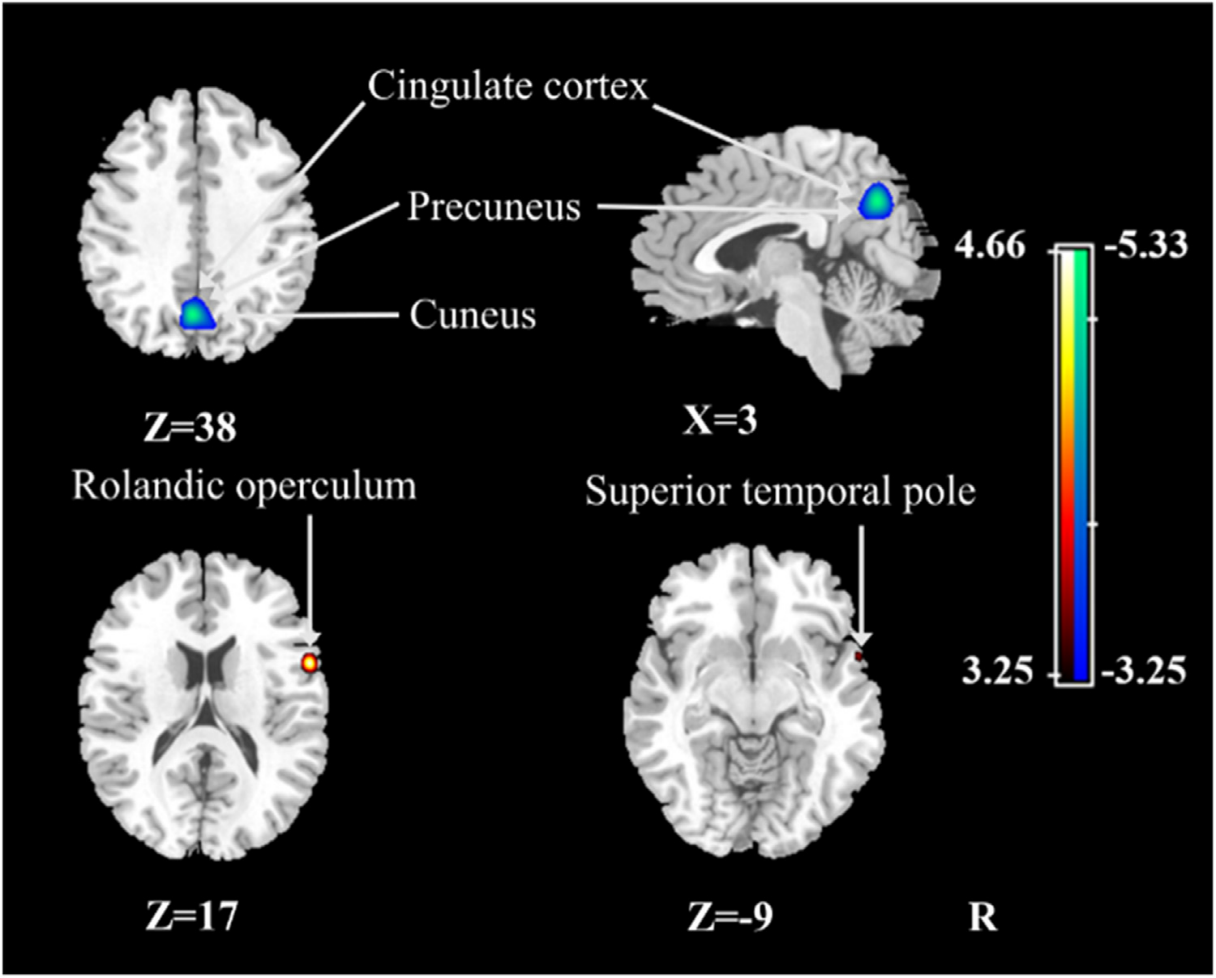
Regional changes in relative CBF after ANCOVA normalization of the global value in patients with MCI due to AD compared with patients with MCI due to non-AD conditions. Cooler colors indicate regions with decreased relative CBF, and warmer colors indicate regions with increased relative CBF in patients with MCI due to AD with respect to patients with MCI due to non-AD conditions. A threshold of 3.25 (*P* < 0.001, uncorrected) was used to overlay the SPM maps onto a standard MRI brain template. CBF, cerebral blood flow; ANCOVA, analysis of covariance; MCI, mild cognitive impairment; AD, Alzheimer's disease; SPM, statistical parametric mapping; MRI, magnetic resonance imaging.
